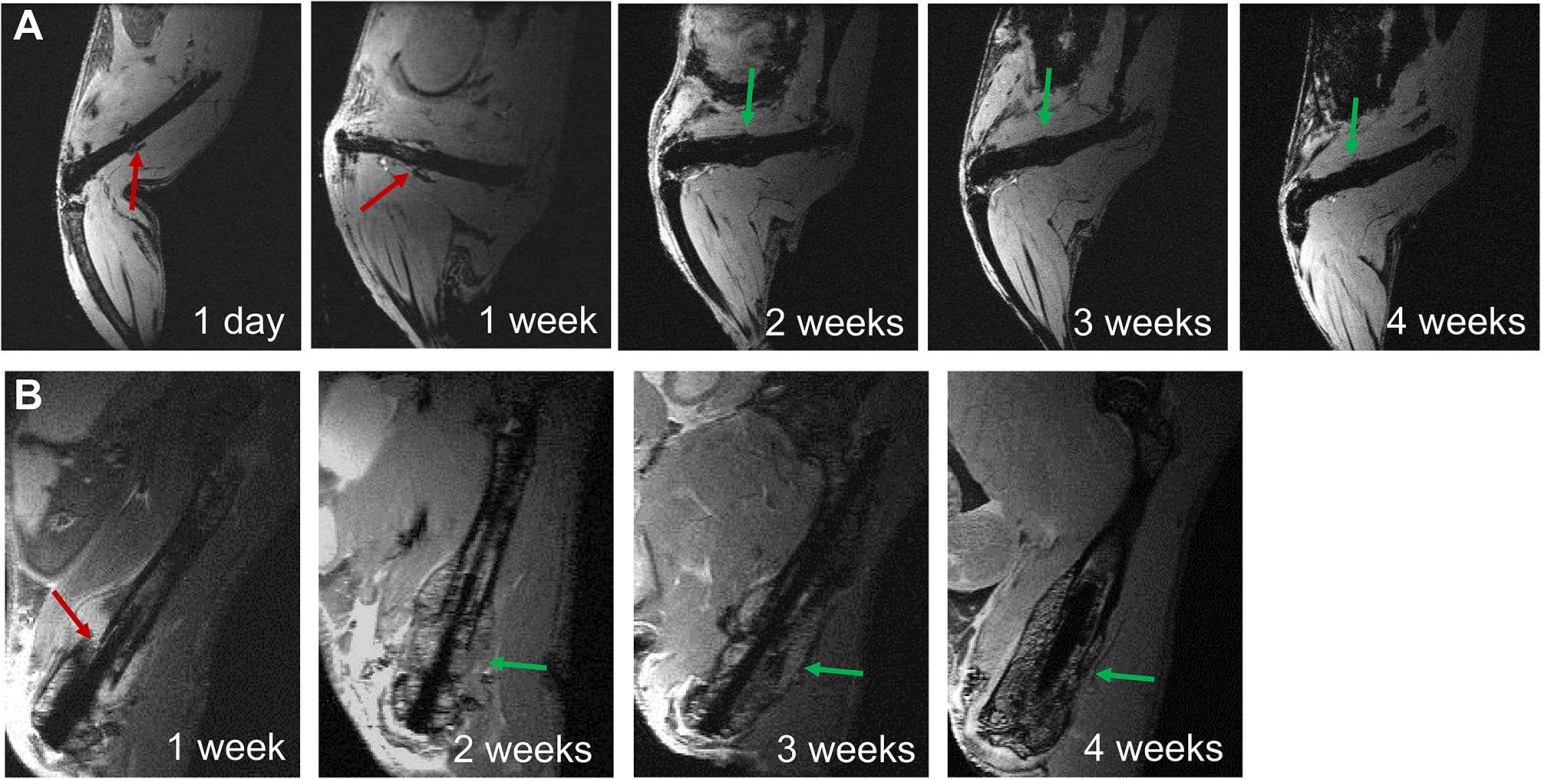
Longitudinal in vivo MRI of fracture healing. 3D T2*-weighted (A, FLASH) and 2D T2-weighted images (B, RARE) showing the fracture site (red arrow) and callus formation (green arrow) over time course.
